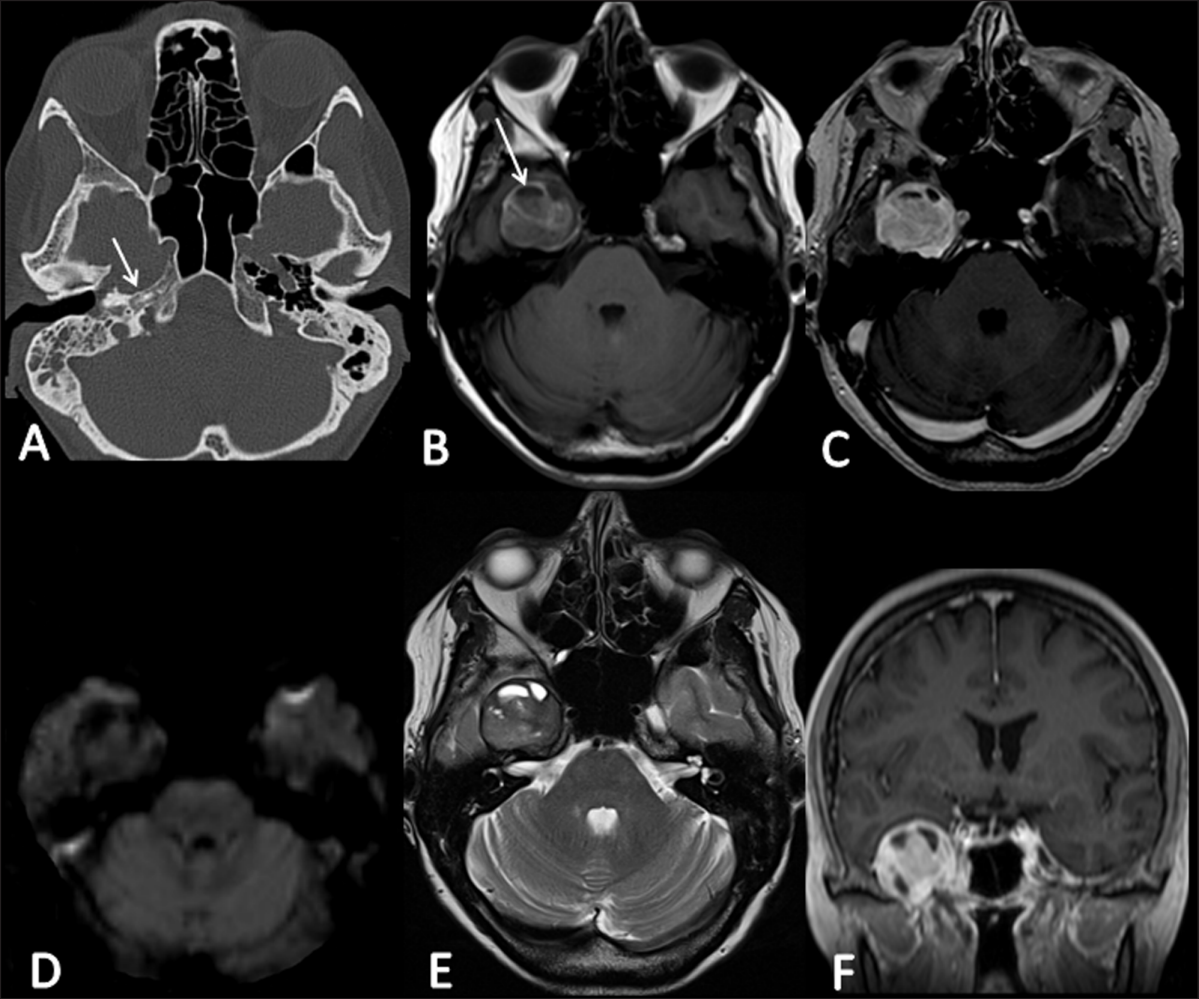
A) CT demonstrates a well-defined petrous lacunar image (arrow). B) T1-weighted image shows the hyperintense round lesion centered on the right Meckel cavum, relying on the intrapetrous carotid artery, and generating a mass effect on the temporal lobe (arrow). D) DWI is negative. E) T2-weighted image shows the presence of cystic areas. C) and F) Axial and coronal T1-weighted images show a slightly heterogeneous enhancement after contrast administration.
